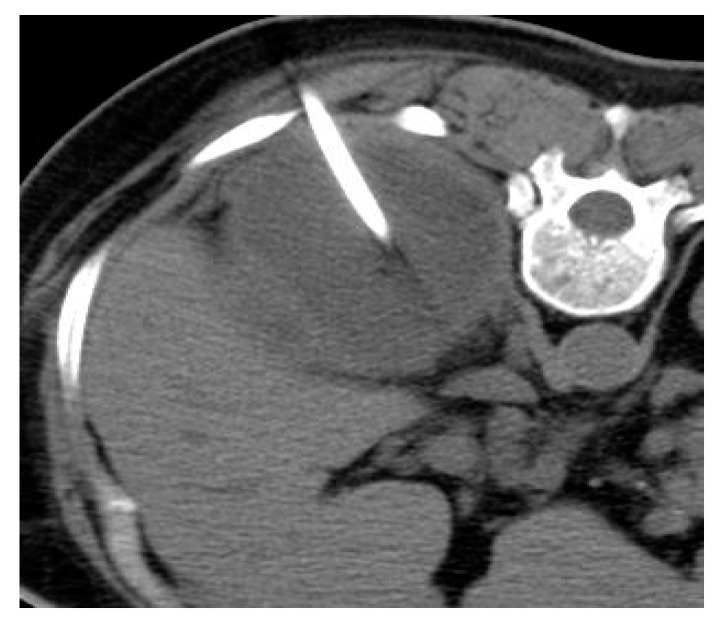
CT guided tube drain of the right renal abscess of the case number 5.
